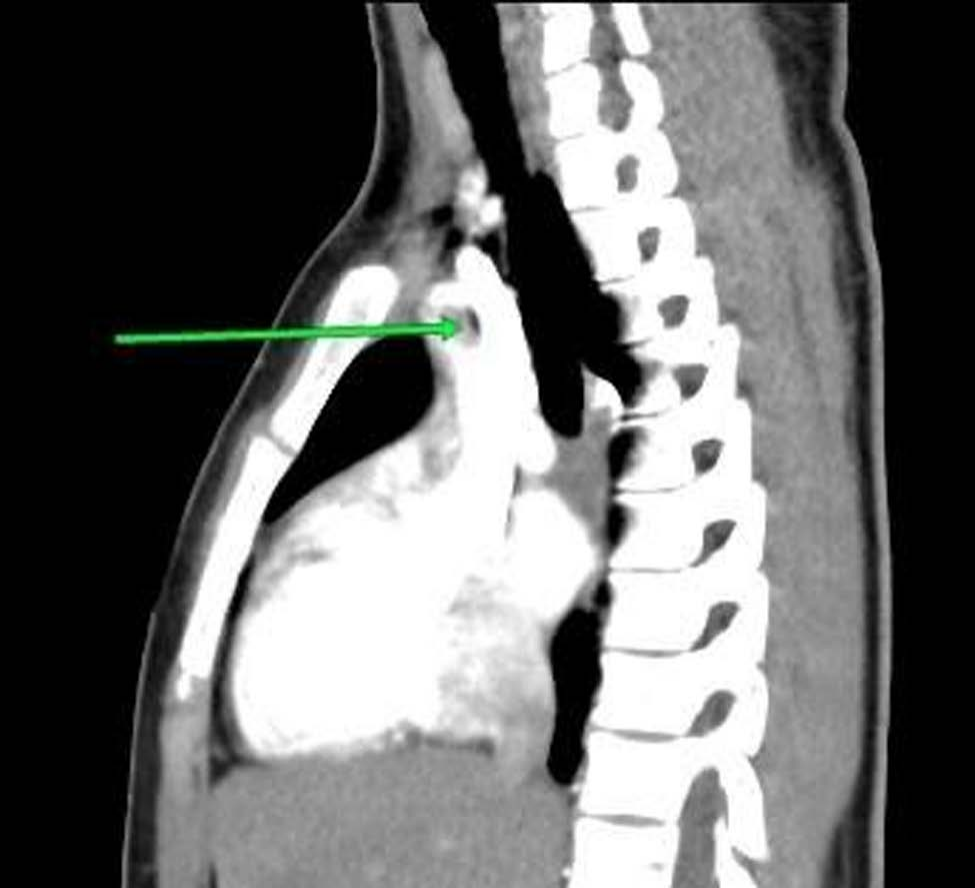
Sagittal section of a computed tomography at the level of the main pulmonary vessels showing a filling defect in the main right pulmonary as highlighted with the arrow.
